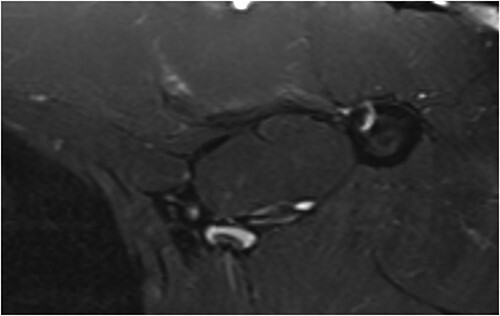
MRI of a patient 1 year post-operatively who underwent reconstruction surgery of the pectoralis major muscle tendon with fascia lata, showing a good image of tendon healing.
